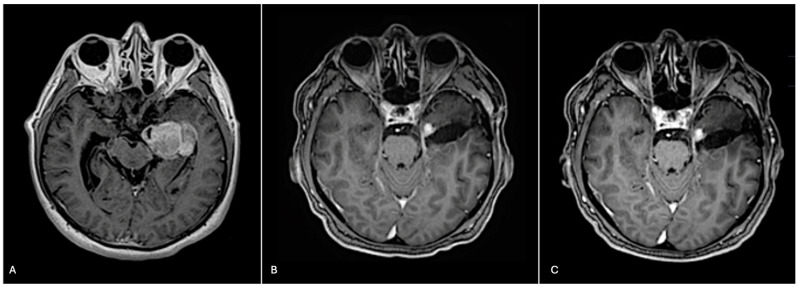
Pre-surgical, post-surgical, and post-2 cycles of temozolomide imaging. (A) Pre-surgical axial T1 brain MRI with contrast reveals a 4.3 cm enhancing mass. (B) Post-radiation axial T1 brain MRI with contrast reveals a residual 1 cm enhancing nodule at the medial aspect of the resection cavity. (C) Following two cycles of adjuvant temozolomide, axial T1 brain MRI with contrast reveals a stable residual 1 cm enhancing nodule at the medial aspect of the resection cavity.
